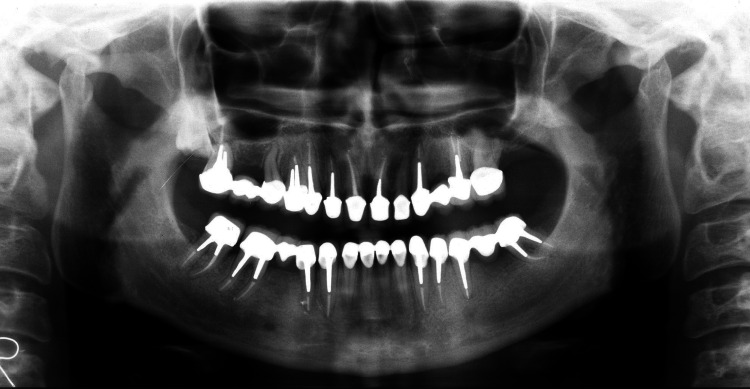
Post-treatment - panoramic X-ray
